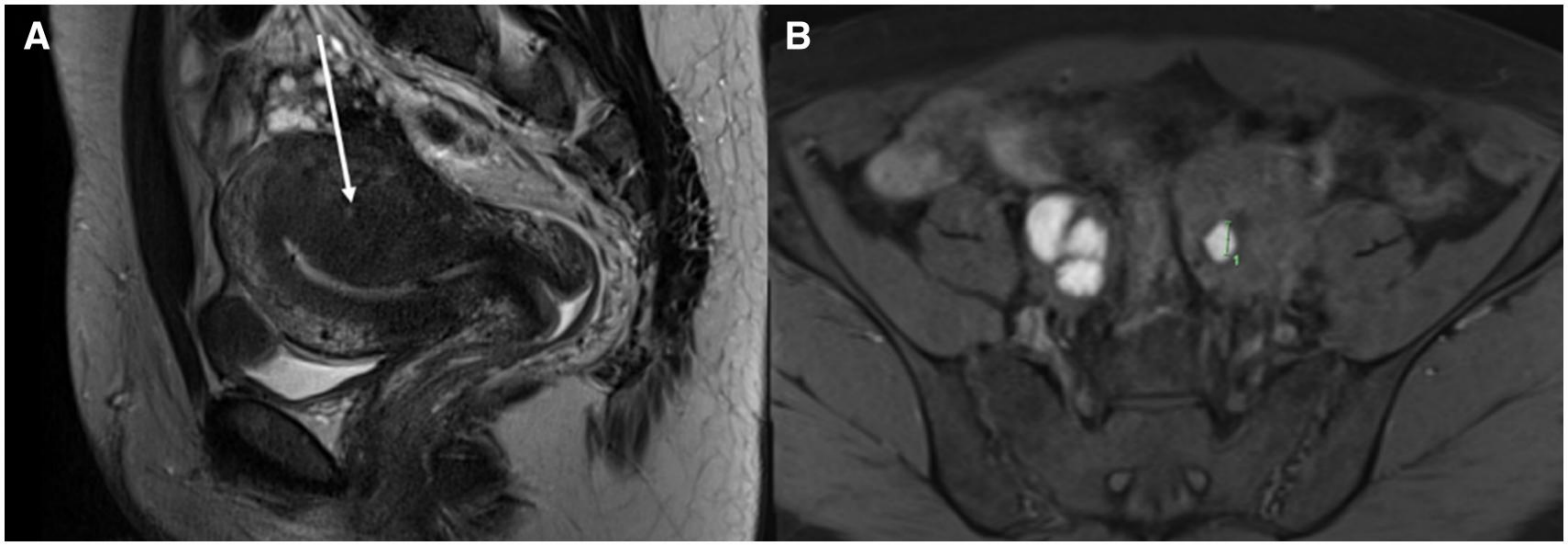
44-year-old female with a history of heavy menstrual bleeding. MRI demonstrated (A) a thickened junctional zone (arrow), in keeping with adenomyosis and (B) bilateral T1-hyperintense ovarian cysts consistent with endometriomas (caliper applied to a unilocular left endometrioma).
